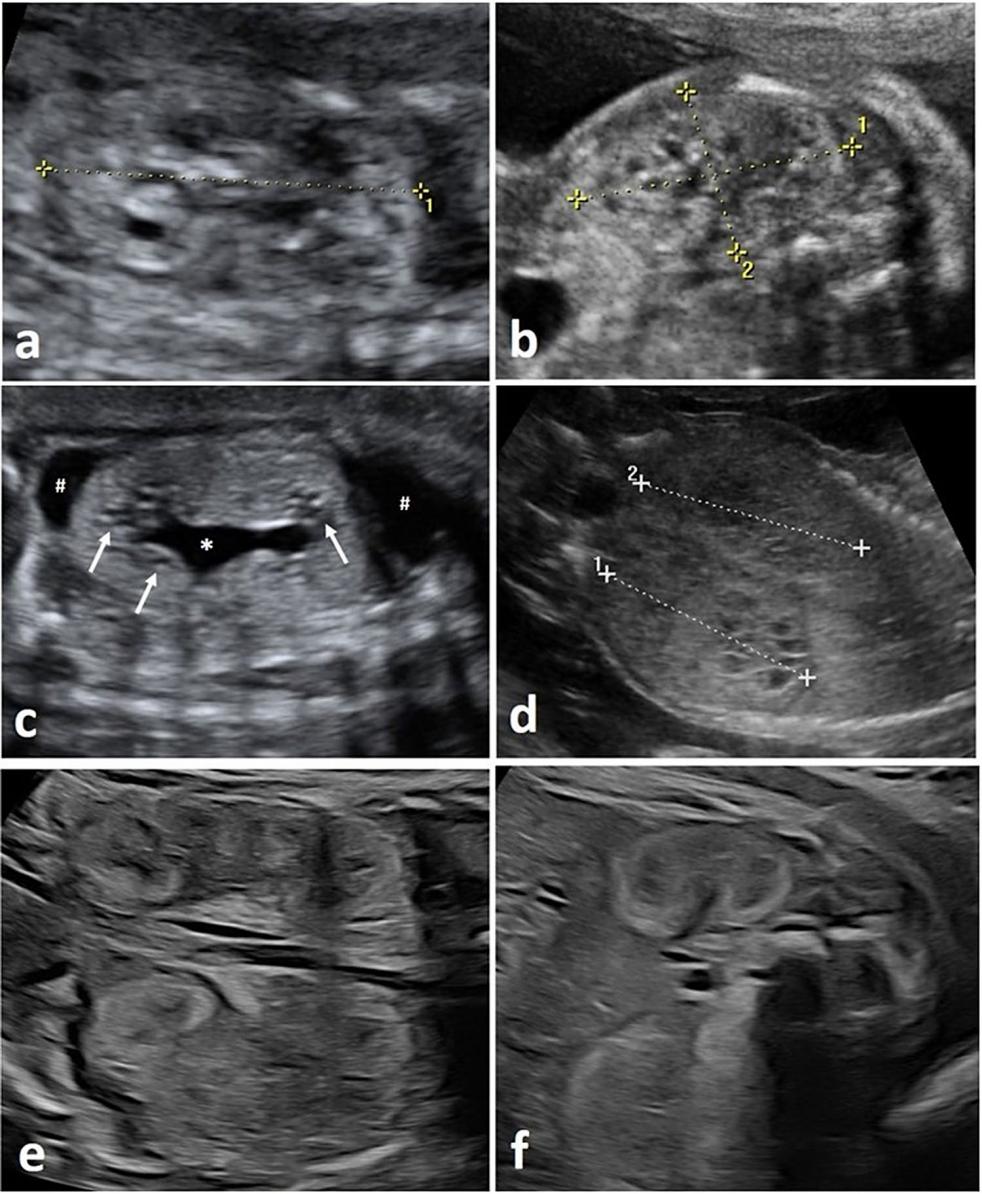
Prenatal ultrasound in fetuses with Joubert syndrome (J*S*), McKusick–Kaufman syndrome/Bardet–Biedl syndrome (*MKKS/BBS*), Jeune syndrome and renal tubular dysgenesis (*RTD*). a+b Show a fetus with JS in 20+6 weeks’: both kidneys present small cysts in both cortex and medulla; c shows a fetus with *MKKS/BBS* at 26+0 weeks’: kidneys are enlarged and hyperechogenic with absent *CMD*, small cysts (white arrows) as well as mild hydronephrosis (asterisk); additionally, this fetus showed mild ascites (number sign); d shows a fetus with Jeune syndrome in 23+2 weeks‘: kidneys present enlarged, isoechogenic with absent *CMD* and small cysts in both cortex and medulla; e+f show fetal kidneys in RTD at 32+1 weeks’: kidneys present enlarged, hyperechogenic and with reduced *CMD*
